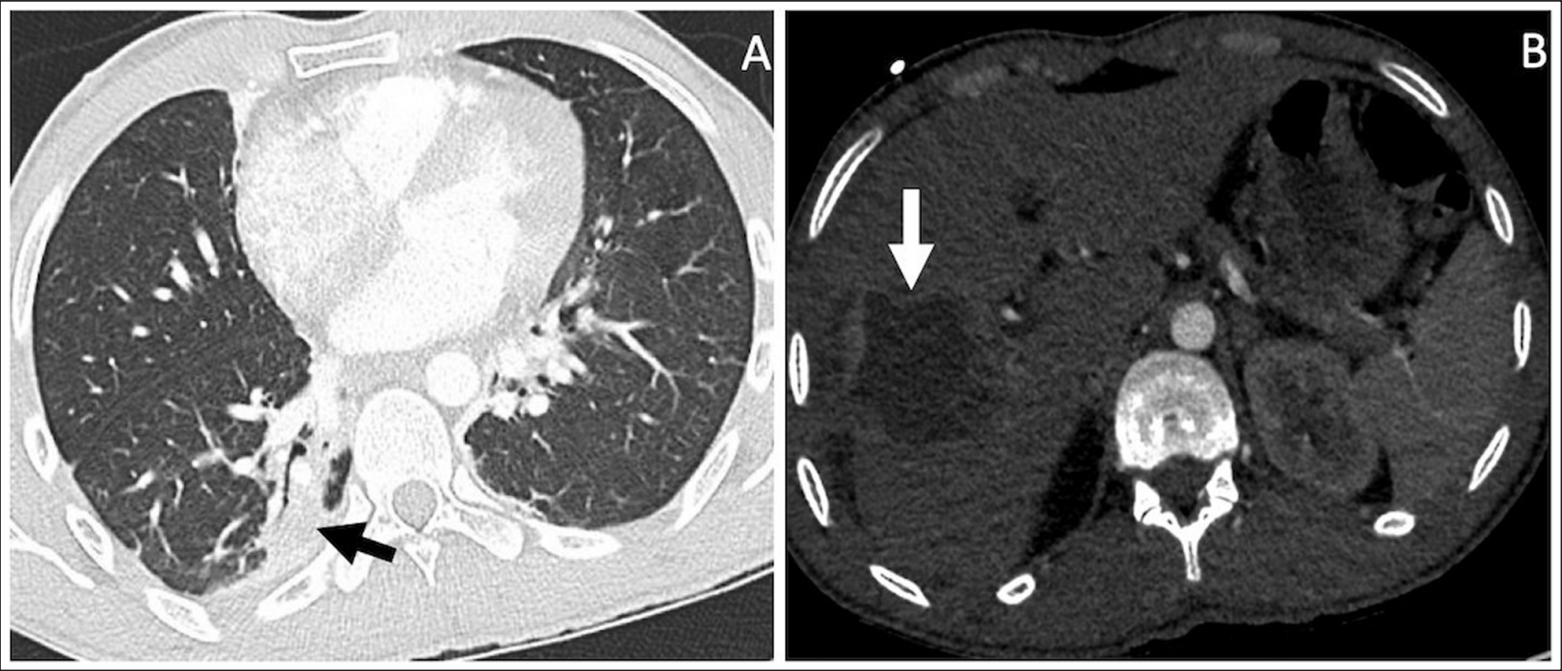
CT pulmonary angiogram (CTA) shows in A a right lower lobe atelectasis (black arrow), and in B a cystic liver lesion (white arrow).
